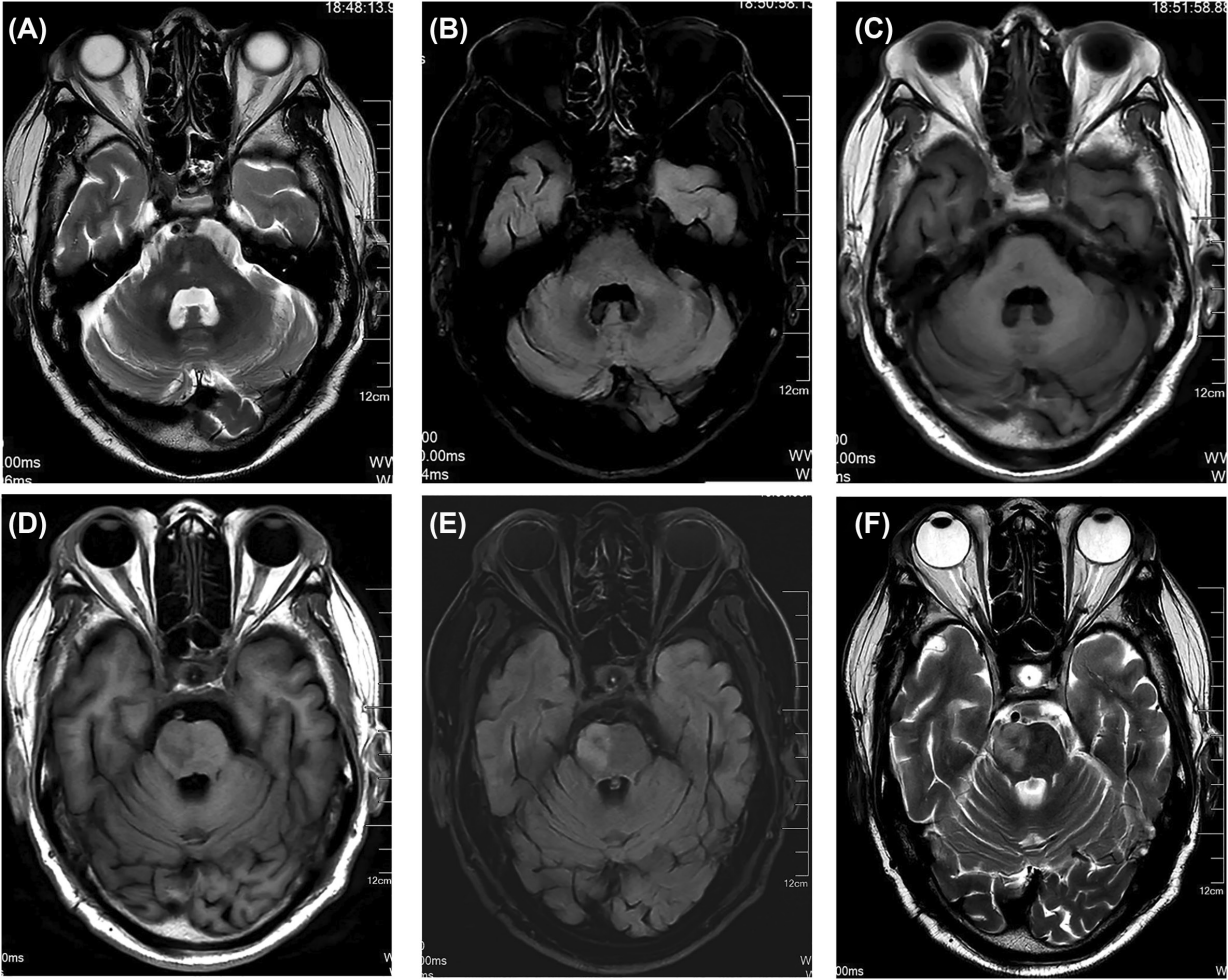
MRI imaging showed bilateral symmetrical wallerian degeneration in the middle cerebellar peduncles. (A–C) Symmetrically hyperintensity on T2/FLAIR WI, with hypointensity on T1 WI in the bilateral middle cerebellar peduncles. Low T1W signal intensities in the right pons, with hyperintensity on FLAIR and T2 WI. (D–F) Low T1W signal intensities in the right pons, with high T2W and FLAIR signal intensities.
